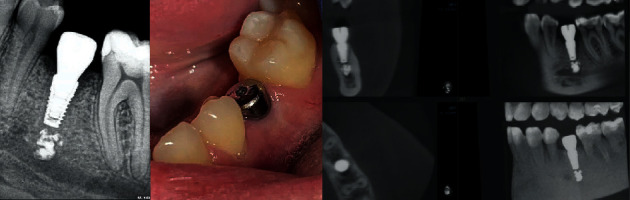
Intraoral X-ray. Condition in the oral cavity with the installation of the gum shaper. CBCT 1 month after implant placement.
